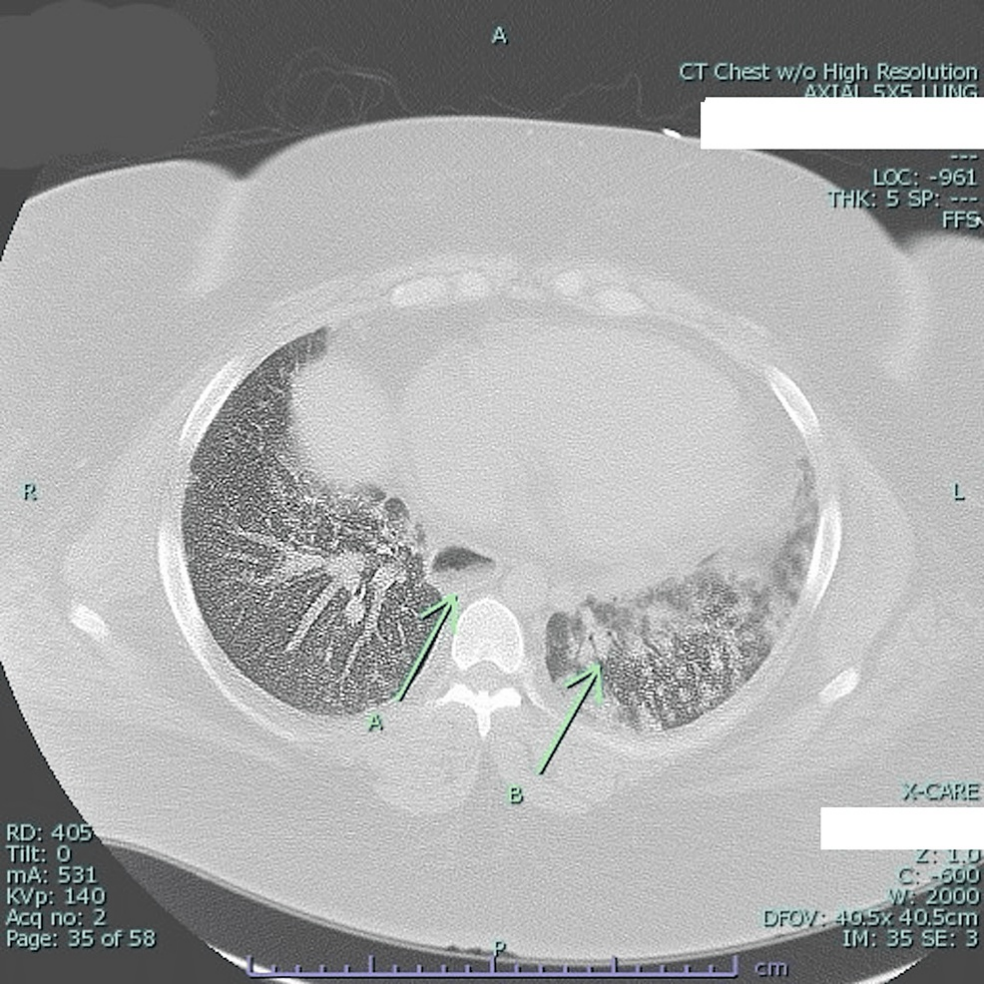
CT of chest without contrast Green arrow A: small amount of debris in distal esophagus; Green arrow B: airspace consolidation with groundglass opacities.
